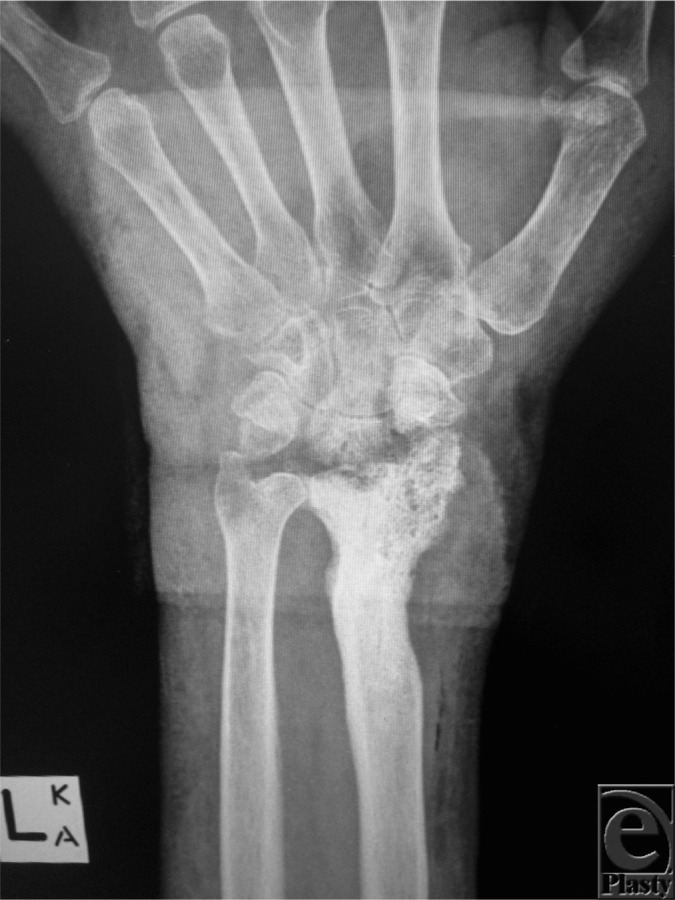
Plain radiograph of the patient's left forearm and wrist demonstrating underlying chronic osteomyelitis. There is evidence of osteolysis, sequestra, involucrum, and permeative changes to the distal radius and the radiocarpal joint.
